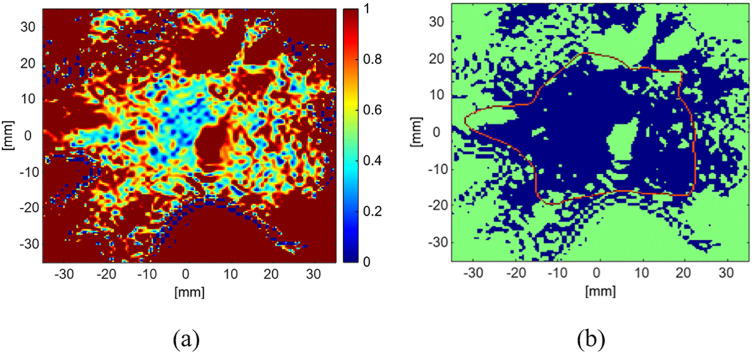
Two-dimensional gamma analysis comparing the TPS-calculated dose distribution and the R2-MRI–derived dose distribution in the central axial slice using a gamma criterion of 3%/3 mm. Panel (a) shows the gamma index map, while panel (b) shows the corresponding satisfactory agreement map, where passing pixels (γ < 1) are displayed in blue. The red contour in panel (b) represents the 20% isodose line of the TPS-calculated maximum dose (8 Gy).
